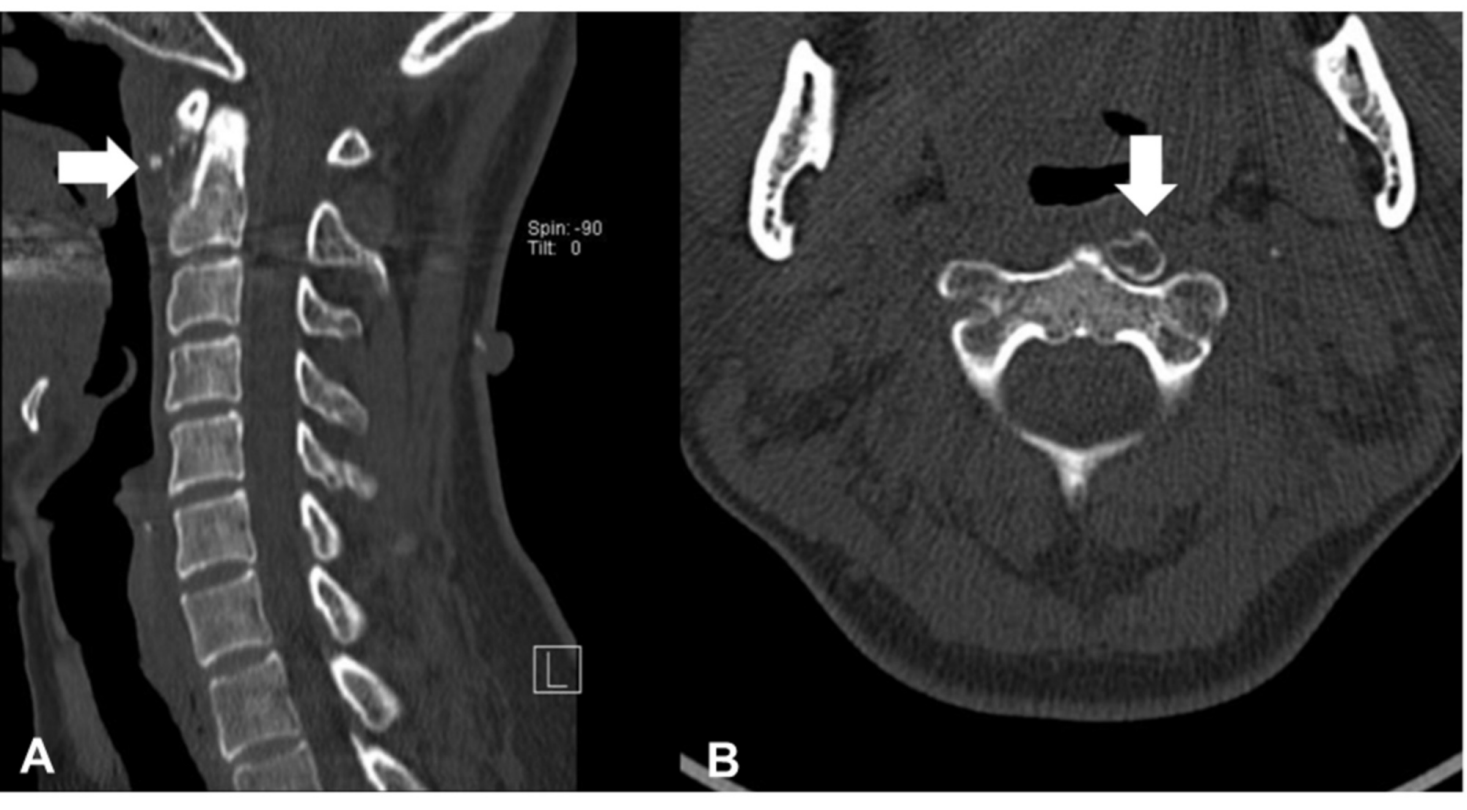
Computed tomography (CT) scans of the cervical spine at the one-month follow-up (A) Sagittal and (B) Axial CT showing marginal calcification, suggesting an eggshell-like calcification with reduced soft tissue swelling (arrows)
